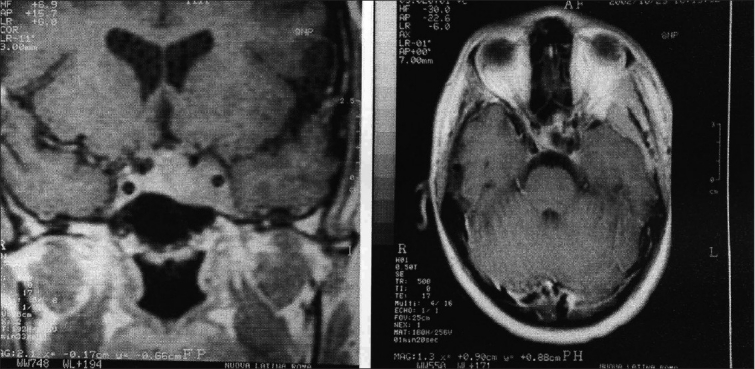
a) MRI, T1 post-contrast coronal scan shows a Knosp 3-4 intracavernous adenoma. b) Postoperative MRI, T1 axial scan. This demonstrates apparent total removal of the tumor
